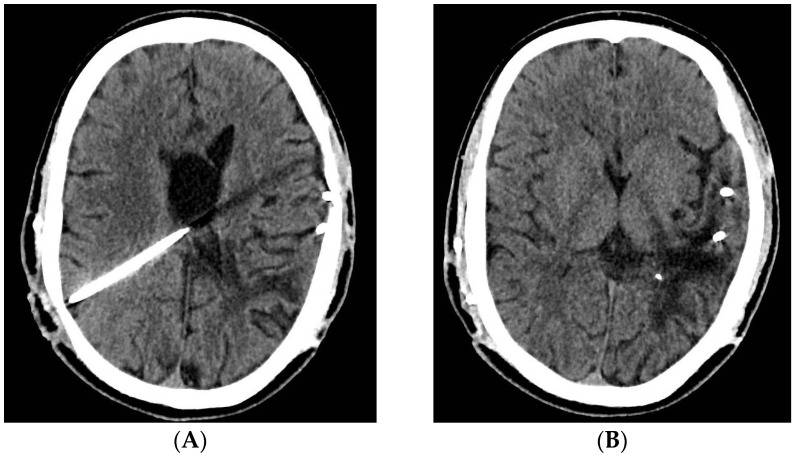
The postoperative aspect of cerebral CT scan—compared to the previous examinations, favorable evolution, with efficient ventricular drainage (A) and dimensional decrease of the ventricular system and the left temporal cystic lesion, indistinguishable from the adjacent left ventricle (B).
